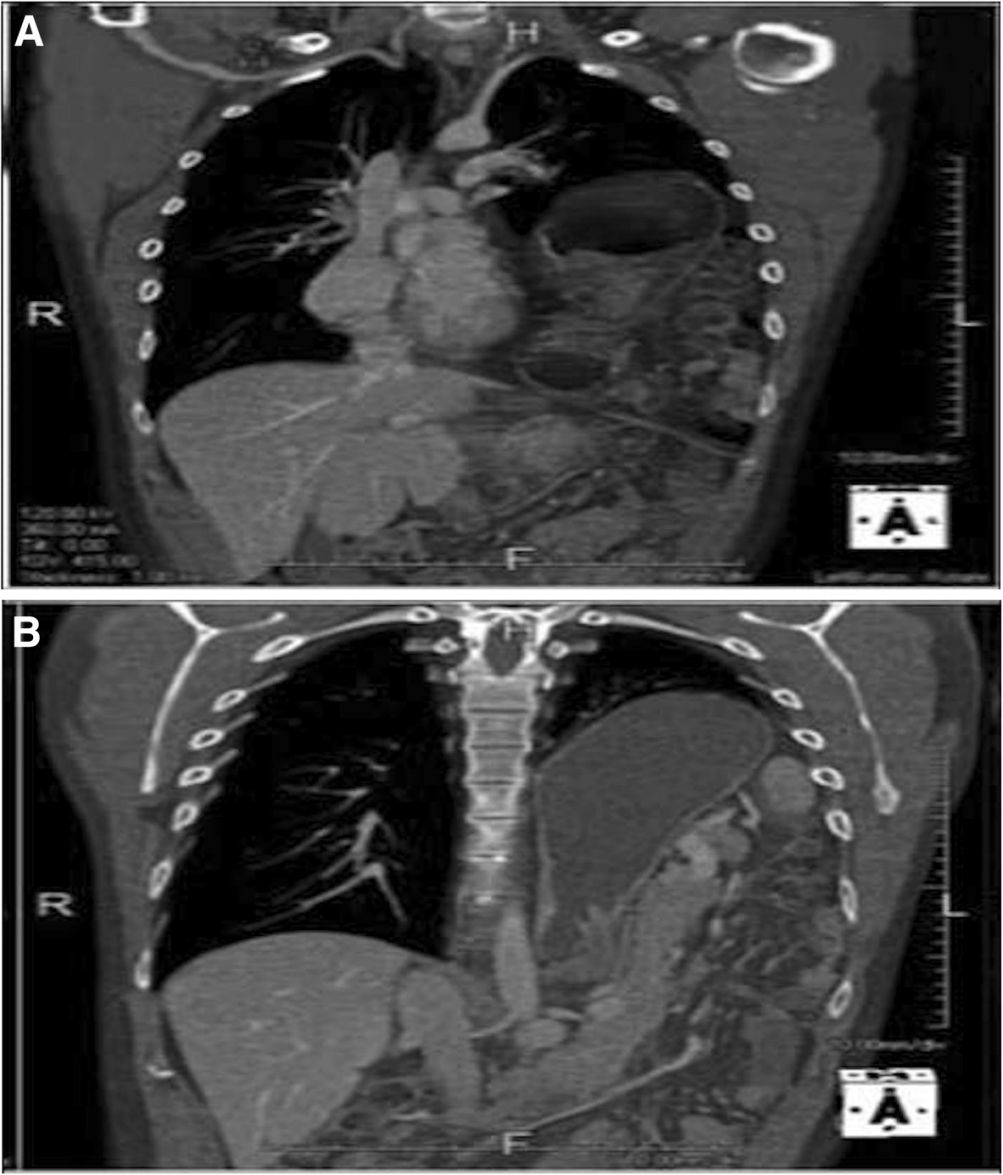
(A,B) Computed tomography scan of the chest. Coronal reconstruction. Part of the stomach, adjacent to the heart (A), is clearly visible. Some bowel loops (B) are also visible on left side of the chest: the left lung is displaced and compressed.
